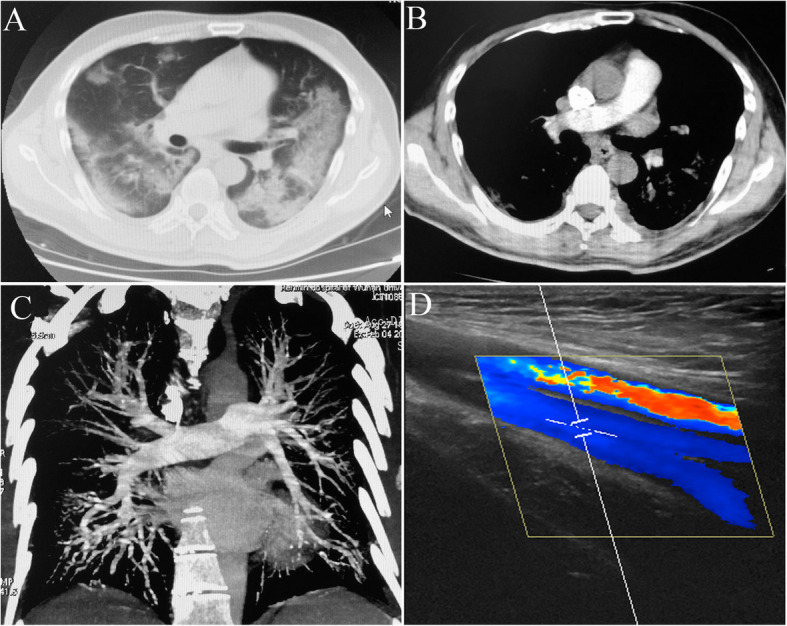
A 59-year-old male diagnosed with COVID-19 who presented with fever, coughing, and hemoptysis. Chest CT upon admission showing ground glass opacities and patchy consolidation (a). He had an elevated D-dimer level of 9.43 mg/L. Wells’ score, Geneva score, and CURB65 score were 7, 7, and 2 respectively. Wells’ score suggested high probability of pulmonary embolism. CT pulmonary angiography (b, c) and Doppler ultrasonography (d) were then carried out and ruled out pulmonary embolism and deep vein thrombosis in the lower extremities
